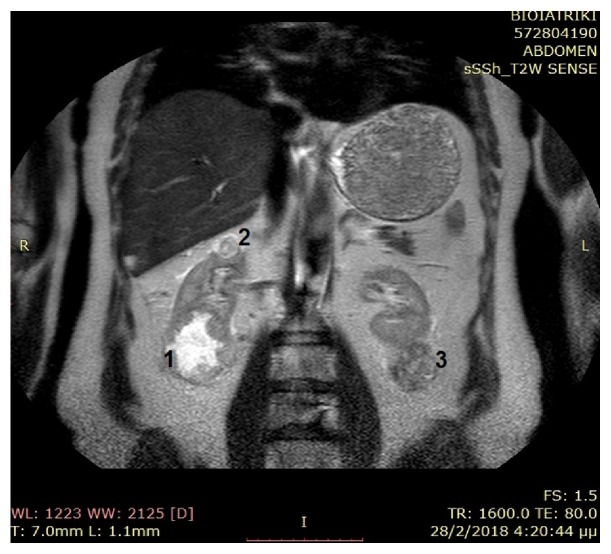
T2-weighted coronal MRI image demonstrating a 6.2x6.3 cm in size, solid mass at the lower pole of the right kidney (1), a 2.4 cm solid mass at the upper pole of the right kidney (2), and a 5.4x4.5 cm in size, solid mass at the lower pole of the left kidney (3).
